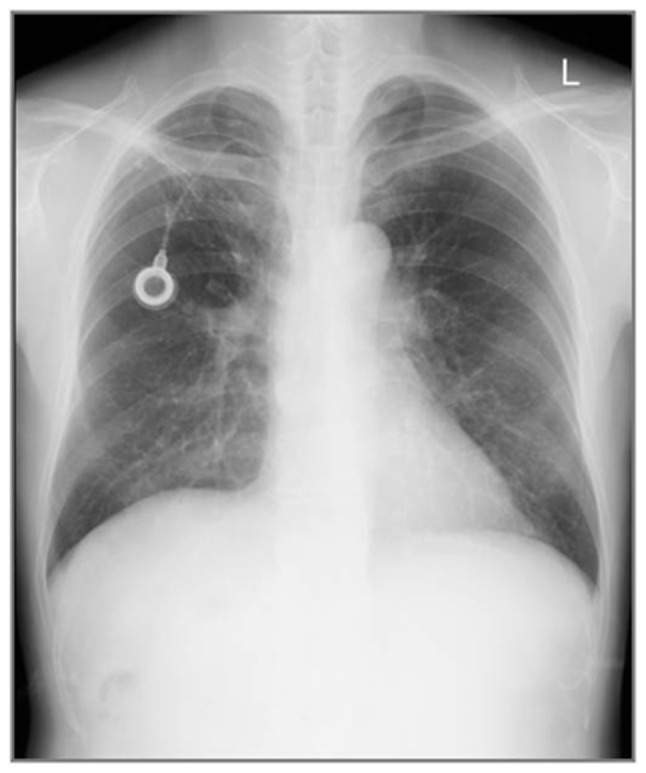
Chest radiograph of the patient in September, 2009 revealed that all metastatic lung nodules had disappeared.
